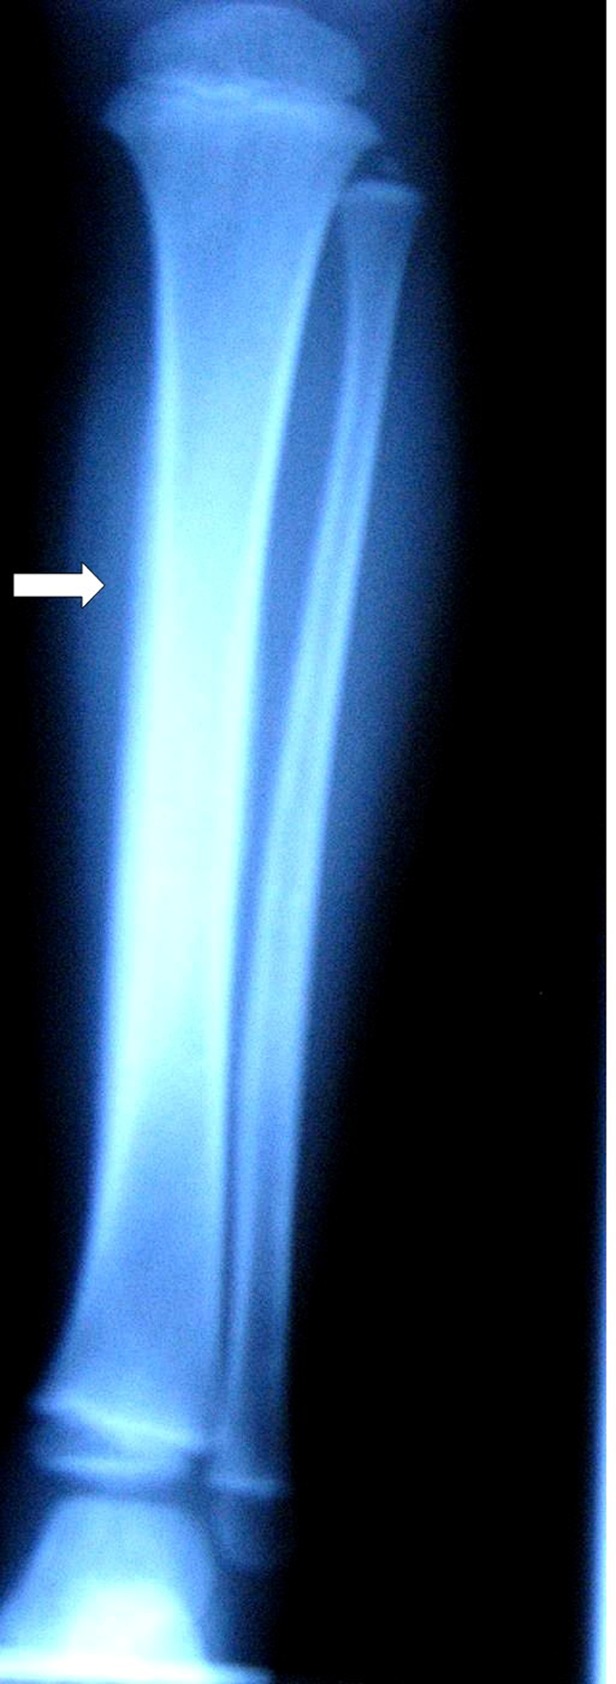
Right leg simple X-ray shows periosteal elevation.
